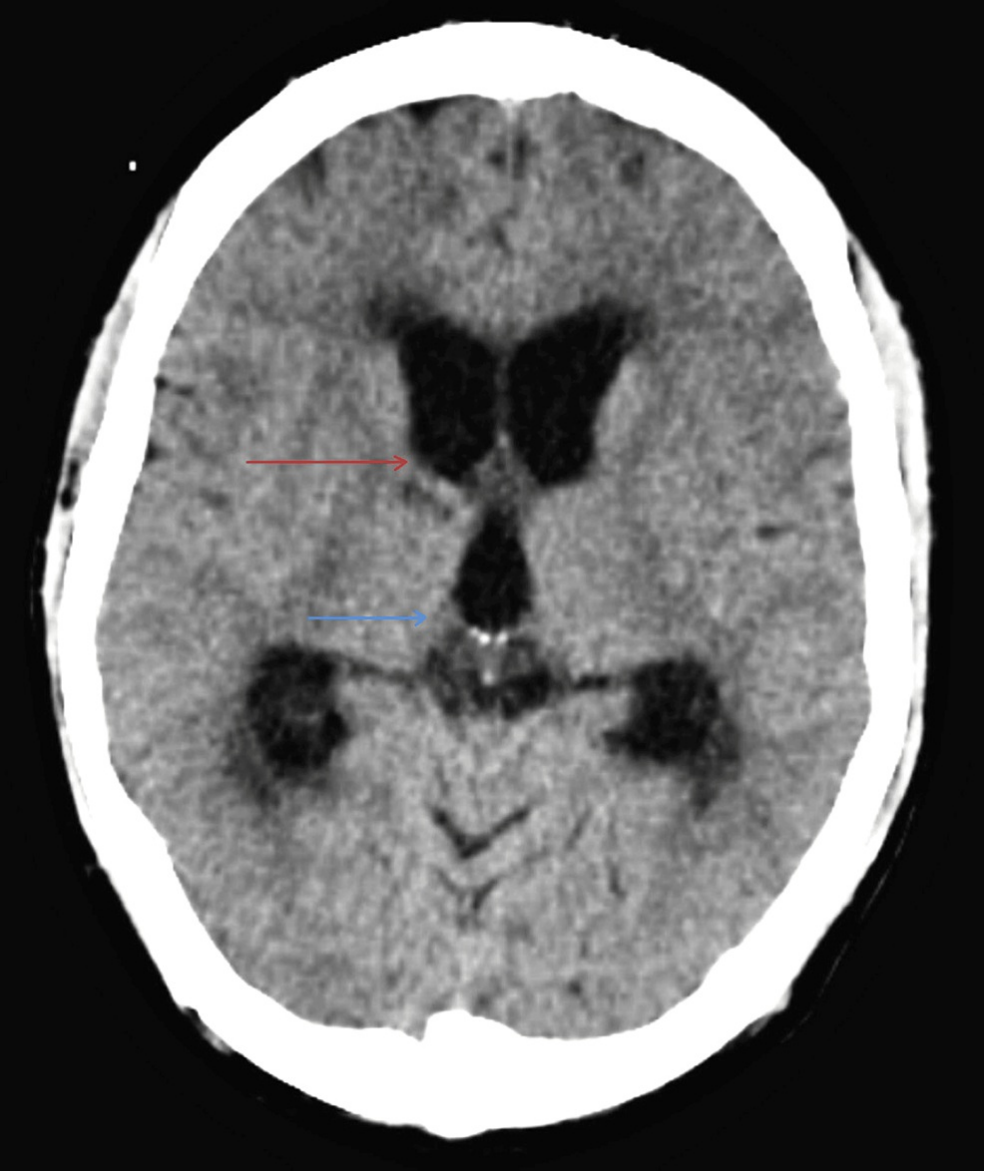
CT brain showing ill-defined hypodense areas in the right caudate nucleus (red arrow) and right thalamus (blue arrow).
